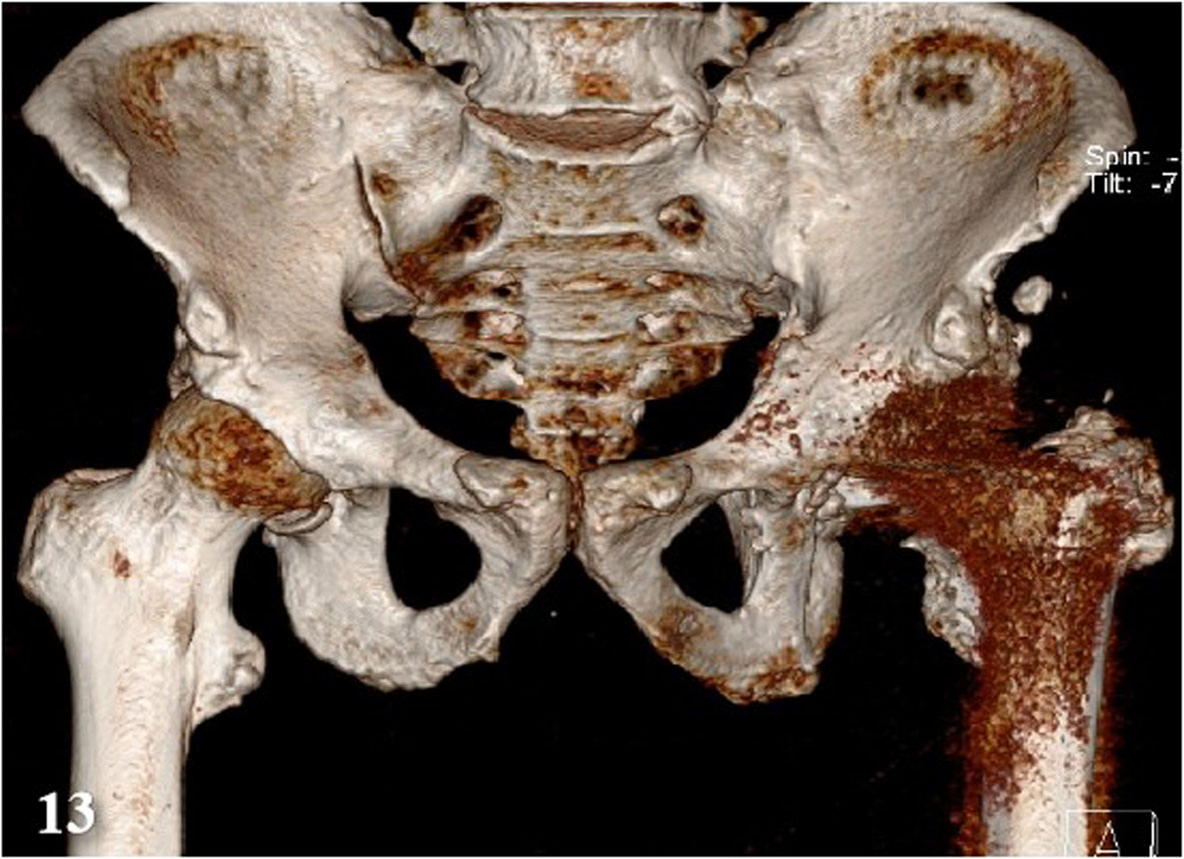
A PJI patient’s 3D CT imaging
